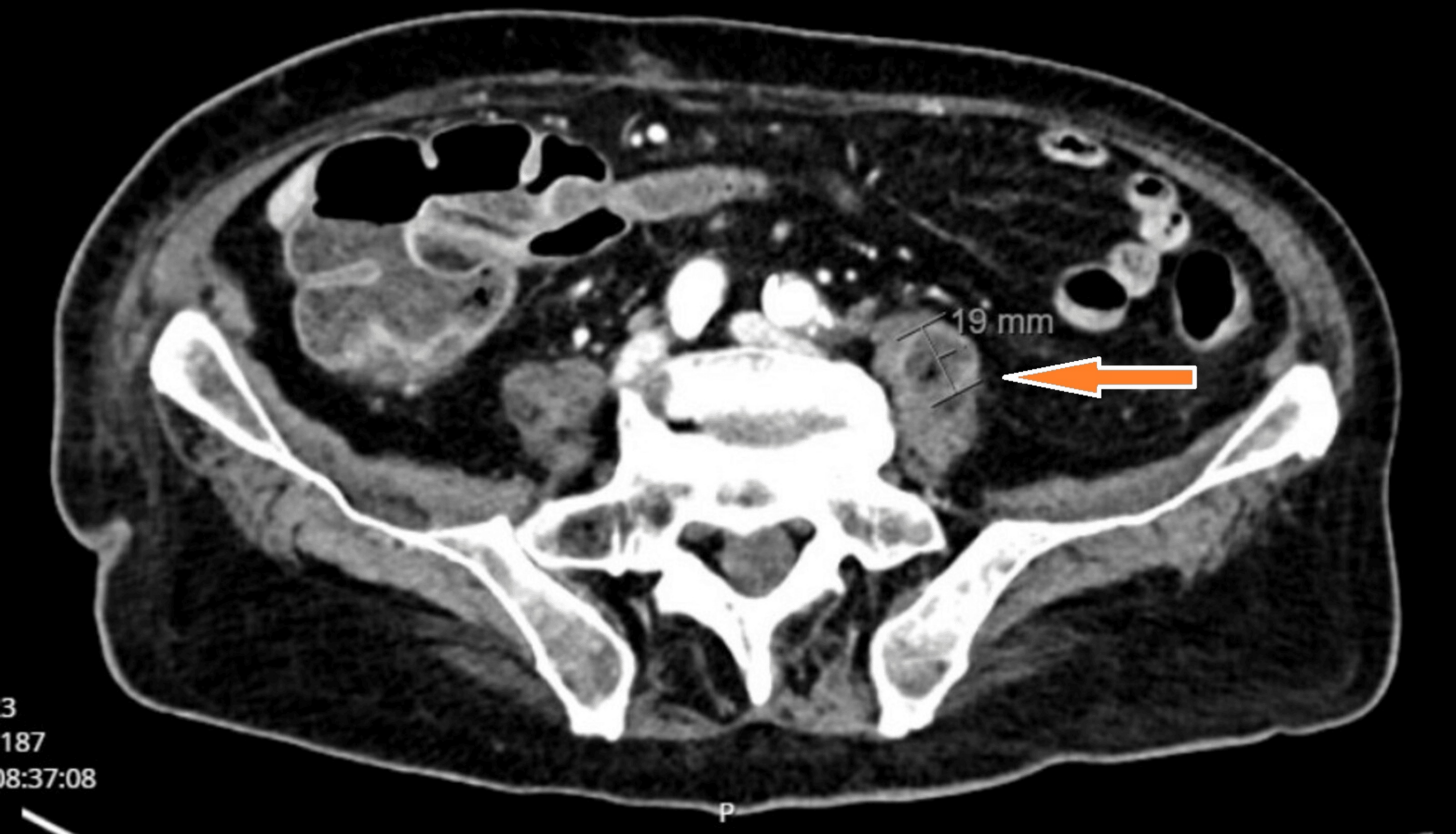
Axial CT scan at the L3/L4 level demonstrating a left-sided psoas abscess adjacent to the affected vertebral bodies. The orange arrow highlights the abscess cavity.
